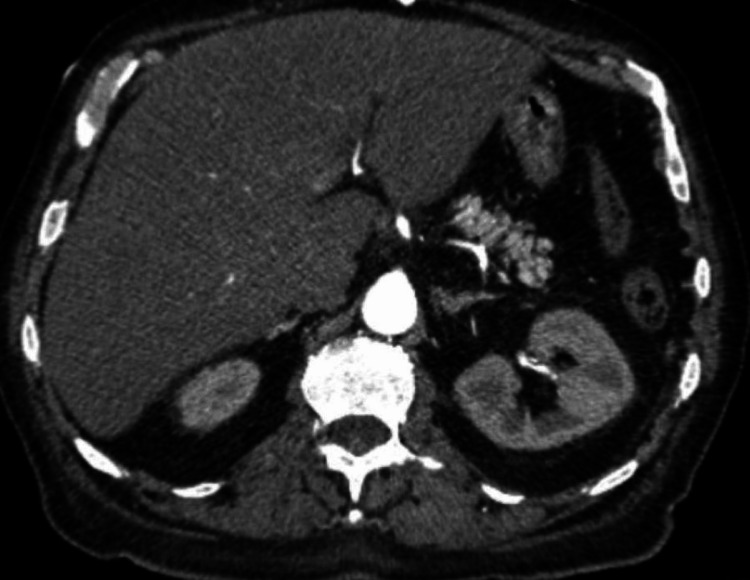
Computed tomography (CT) scan of the abdomen showing normal liver parenchyma and size.
